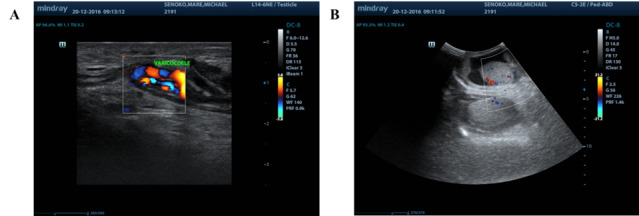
Ultrasound of the left scrotum
